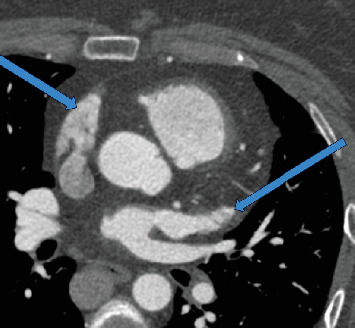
Coronal CTA demonstrates long and narrow bilateral atrial appendages suggesting left isomerism (blue arrows).
